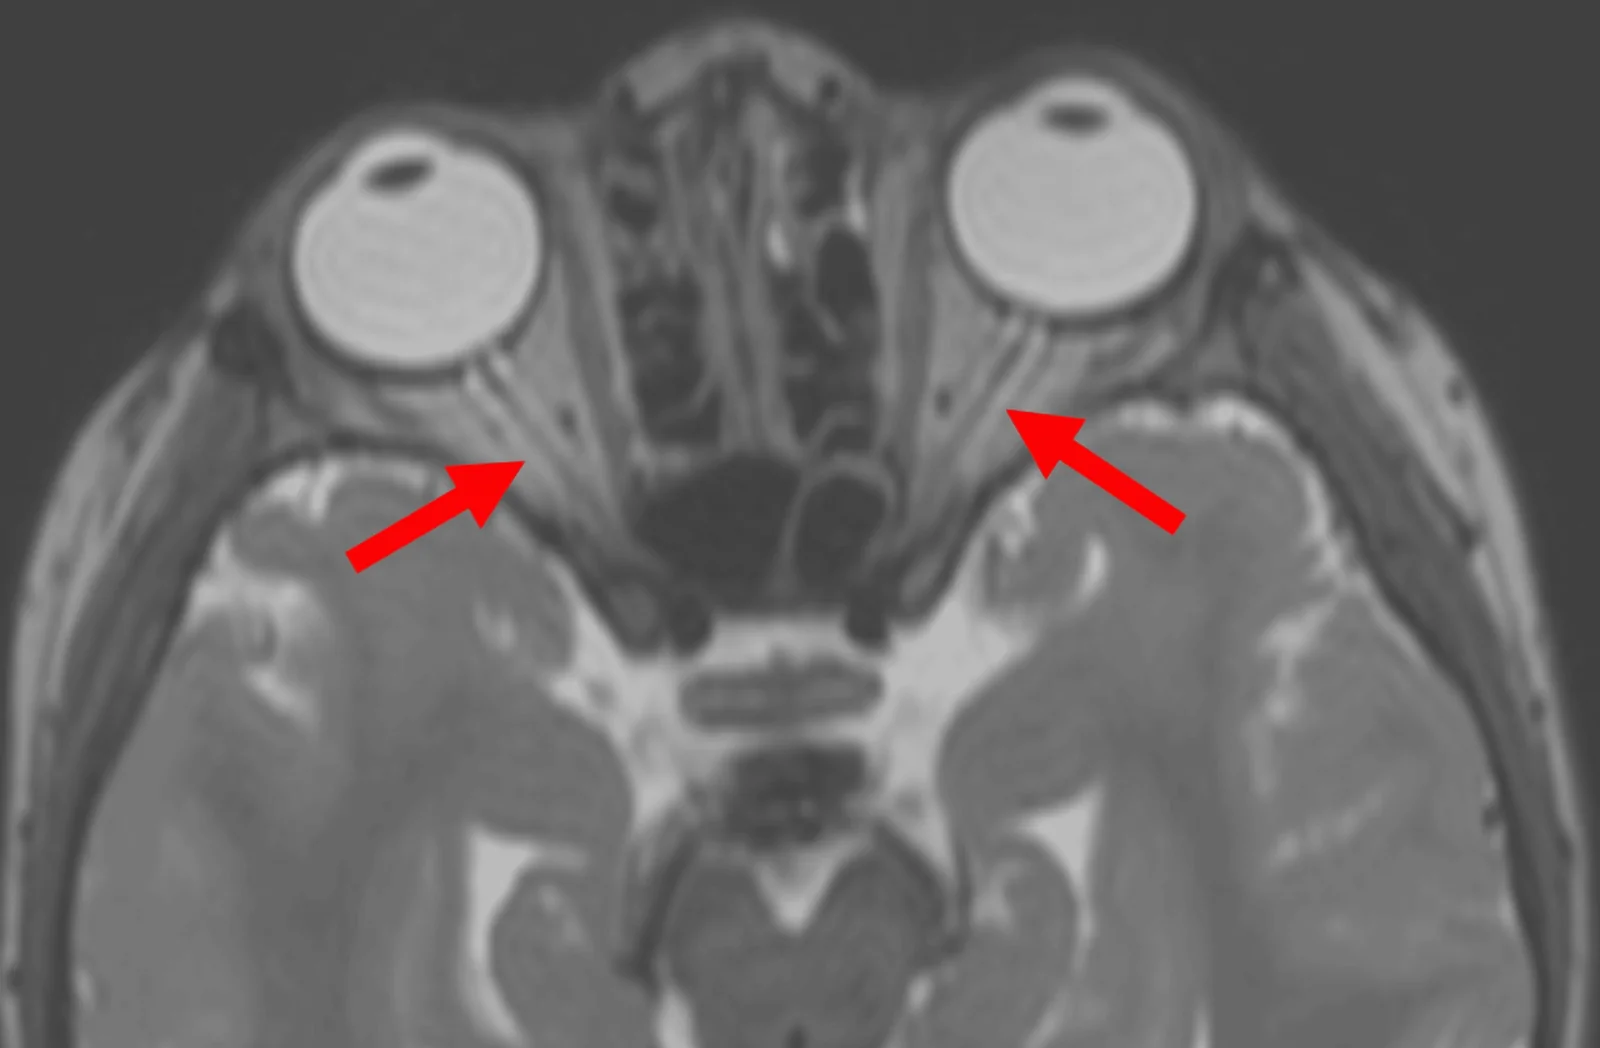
MRI image demonstrating bilateral optic nerve hypoplasia Axial short tau inversion recovery (STIR) MRI image of the patient shows bilateral hypoplastic optic nerves (red arrows).
